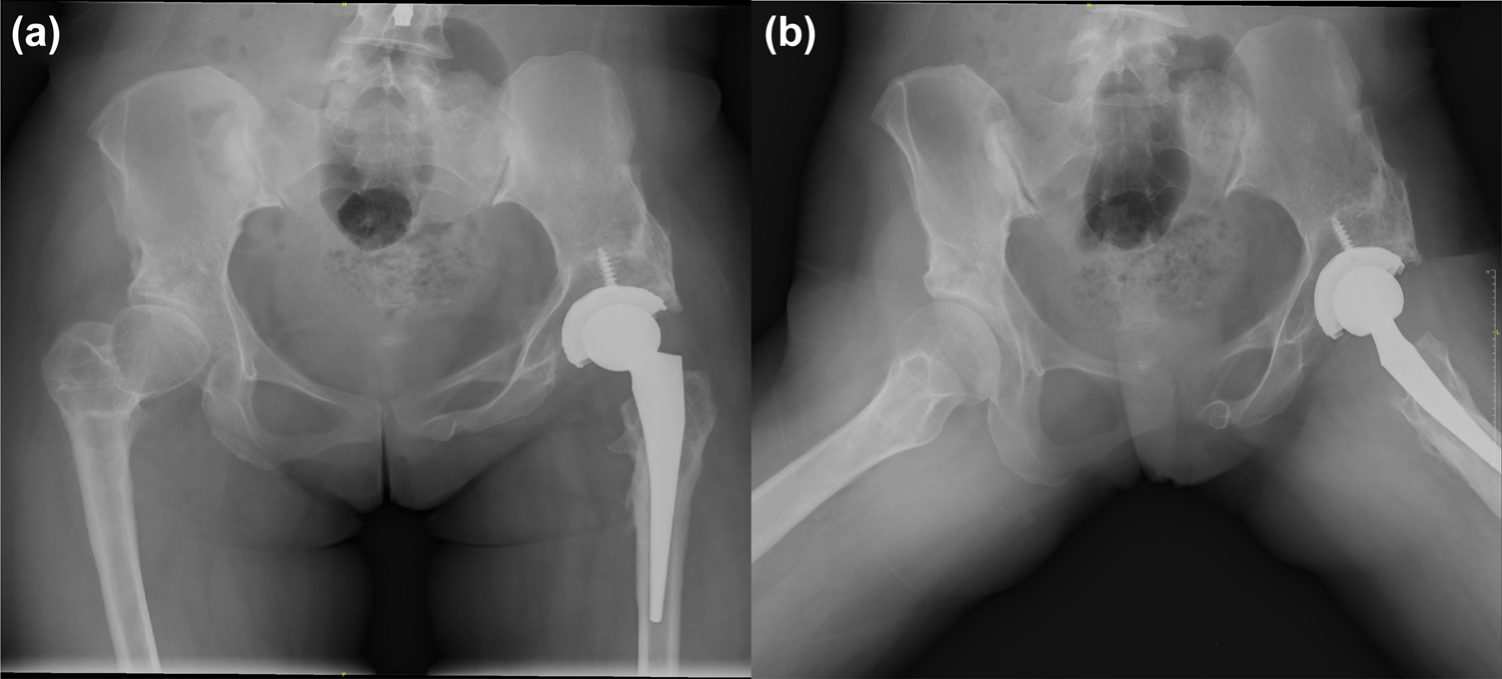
The X-rays of the case in the postoperative 3 months. (a) The anteroposterior radiography. (b) The frog-leg lateral radiography.
